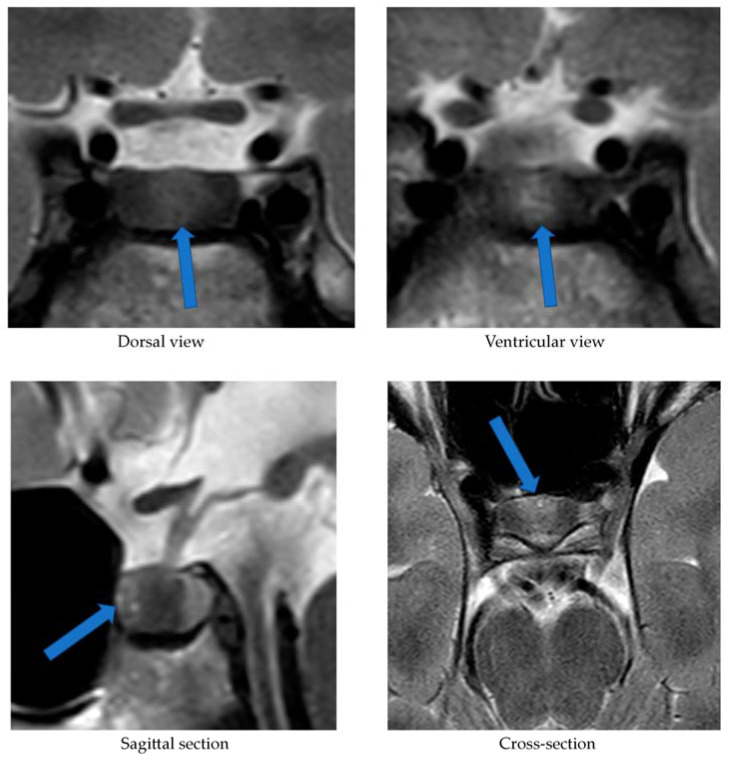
MRI examination image depicting the poorly demarcated area in the anterior part of the glandular lobe. The arrows point to a structure suspected of being an adenoma.
